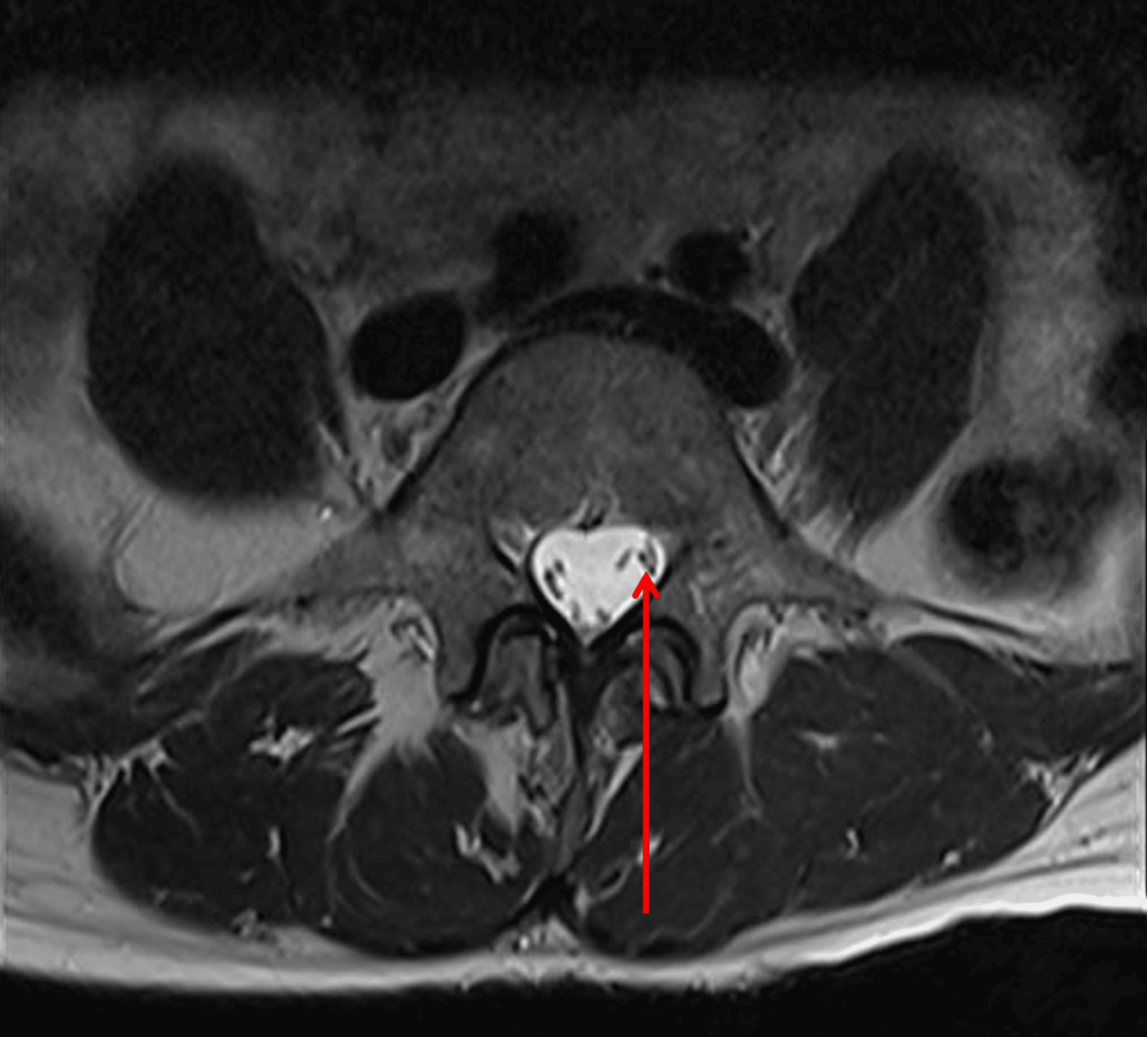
Axial T2-weighted MRI of the lumbar spine at L5. The red arrow depicts the duplicate exiting L5 nerve root.
